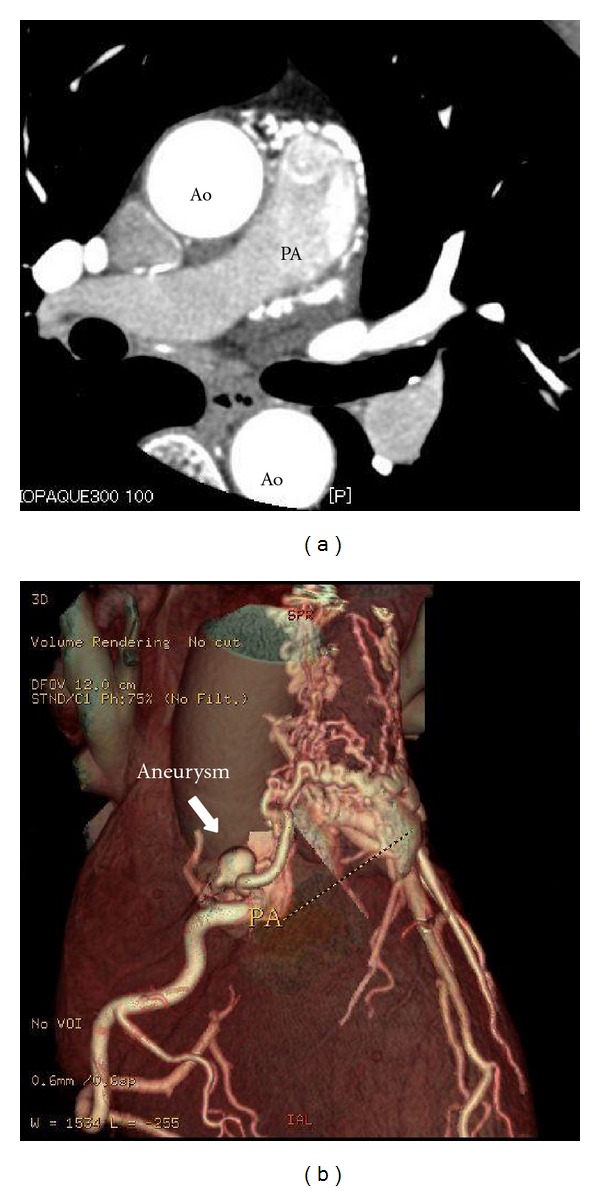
Coronary computed tomography. An axial section of the computed tomography (CT) image revealed dilated vessels around the pulmonary artery PA (a). Three-dimensional CT revealed a saccular aneurysm (arrow), 1 cm in diameter, around the right coronary artery (RCA), and fistula vessels originating from the proximal RCA and left anterior descending artery joined the pulmonary trunk (b).
